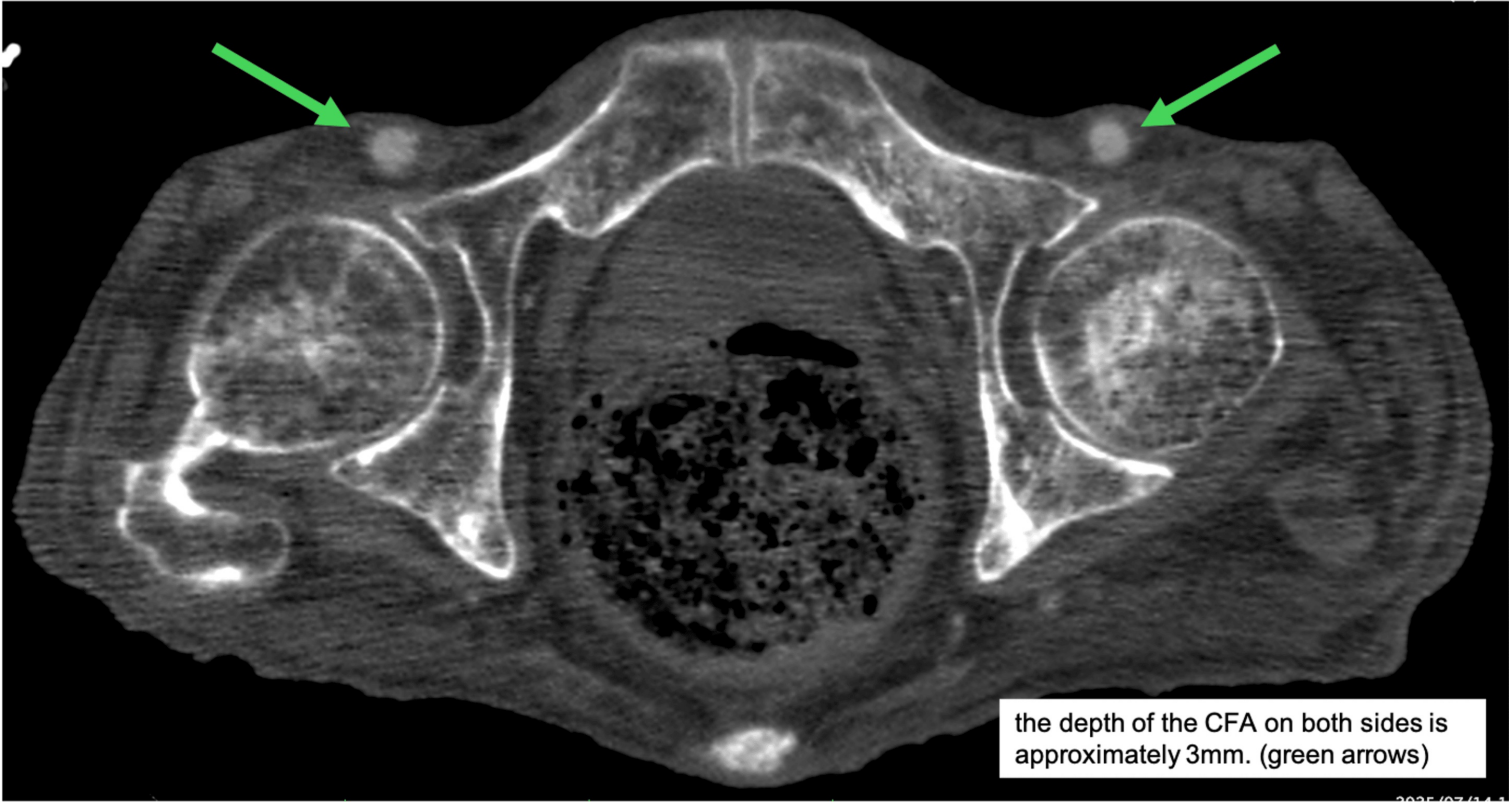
The contrast-enhanced CT scan shows that both CFAs are very shallow.
